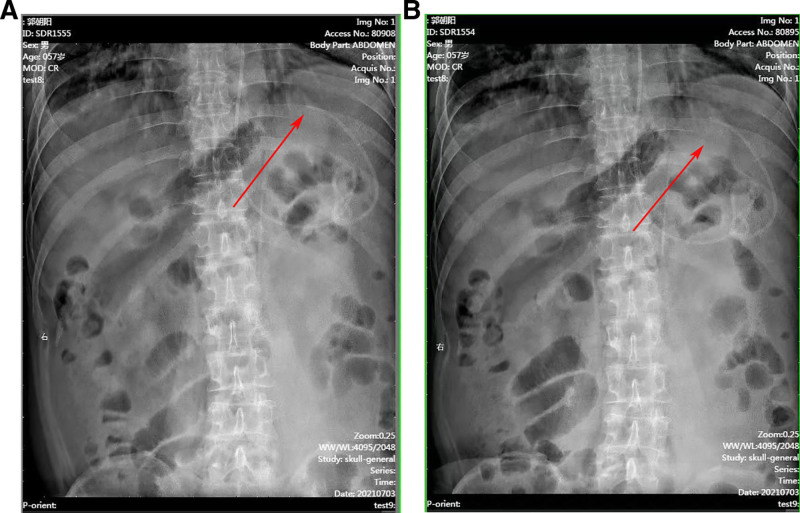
X-ray images of the peritoneal catheter position of a VP shunt at 2 different time points (A and B). The images show no change in the position of the peritoneal catheter between the 2 X-rays. Arrows indicate the peritoneal catheter tip position. VP = ventriculoperitoneal.
